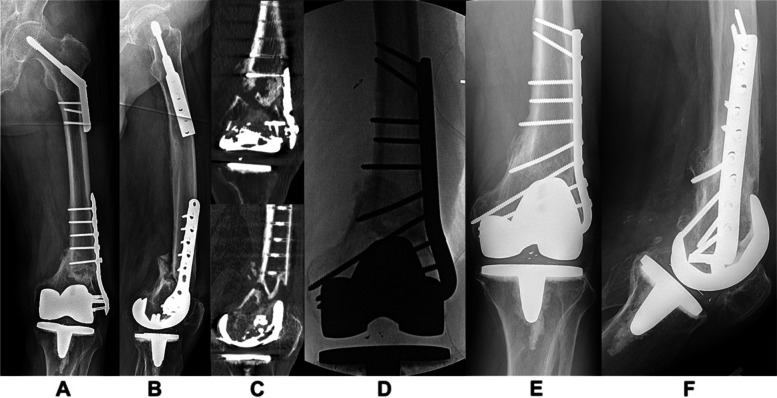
An 85-year-old male fell approximately 8.5 years following a left-sided total knee arthroplasty (and was also 9 years status-post left-sided hip fracture surgery). He sustained a supracondylar periprosthetic distal femur fracture and was surgically treated at a local hospital with a distal femur locking compression plate (LCP). He developed a nonunion and subsequently was treated with a bone stimulator. He presented to our institution 14 months following surgery. Anteroposterior (AP) and lateral imaging revealed a distal femur nonunion in varus deformity with posterior translation a flexion deformity (A, B). CT scan imaging further demonstrated the nonunion morphology (C). Revision open reduction and internal fixation (ORIF) was performed with LCP and screw removal, debridement of the nonunion site, (five) cultures taken, correction of deformity with placement of a 95^0^ blade plate and screws, demineralized bone matrix and bone morphogenic protein-2 (D). He returned for routine follow-up and healed 10 months following ORIF. Most recent follow-up AP and lateral radiographs at 20 months (E, F) revealed a healed nonunion in acceptable alignment and with maintenance of fixation and resumed his activities of daily living
